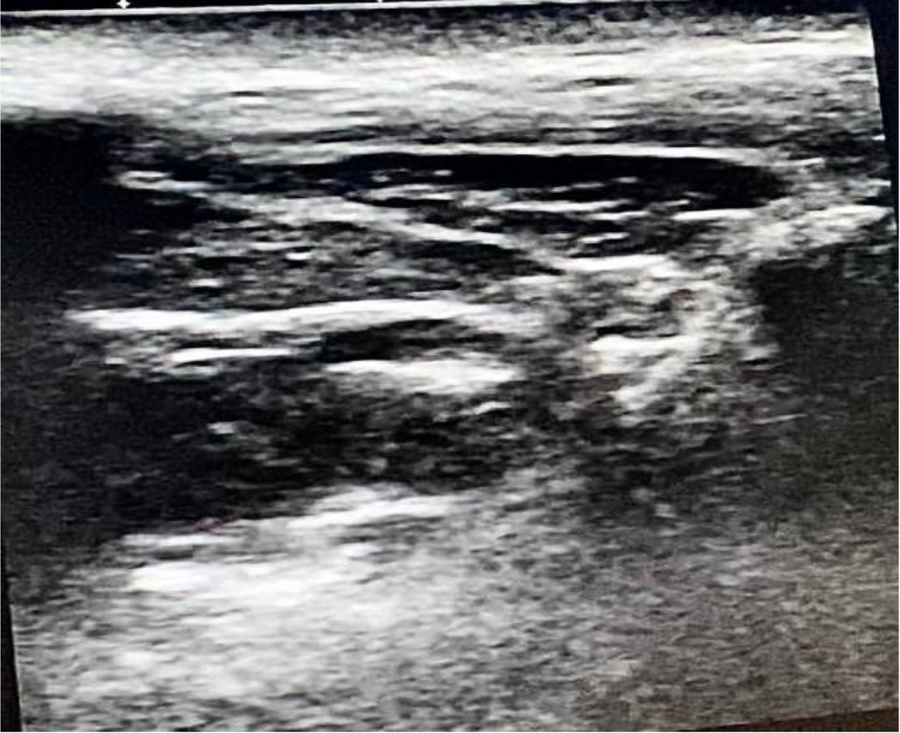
Ultrasound imaging for the PPF after injection of the local anaesthetics
